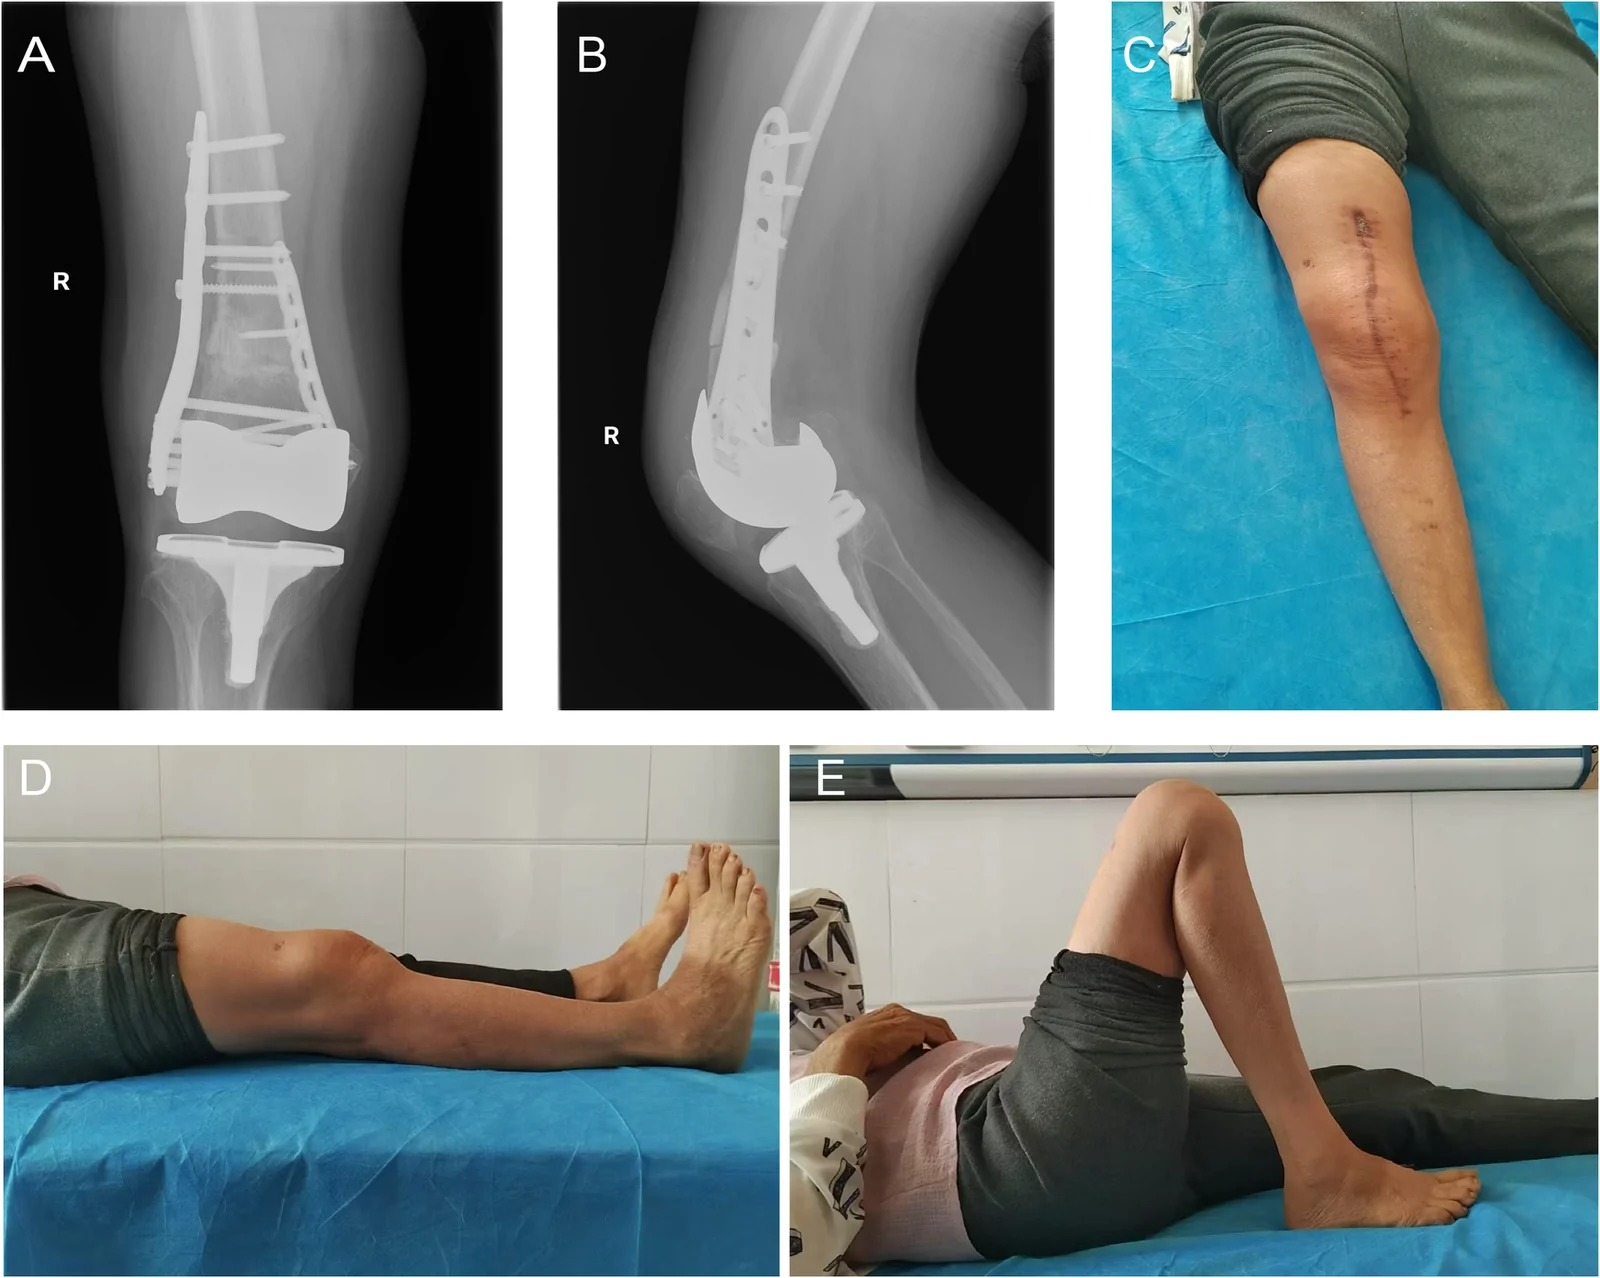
Radiographic and functional findings at 5 months (A,B) radiographs showing maintained prosthetic and plate fixation with progressive bridging and union of the osteotomy. (C) Healed incision without signs of infection. (D,E) Active extension and flexion of 0°−135°.
